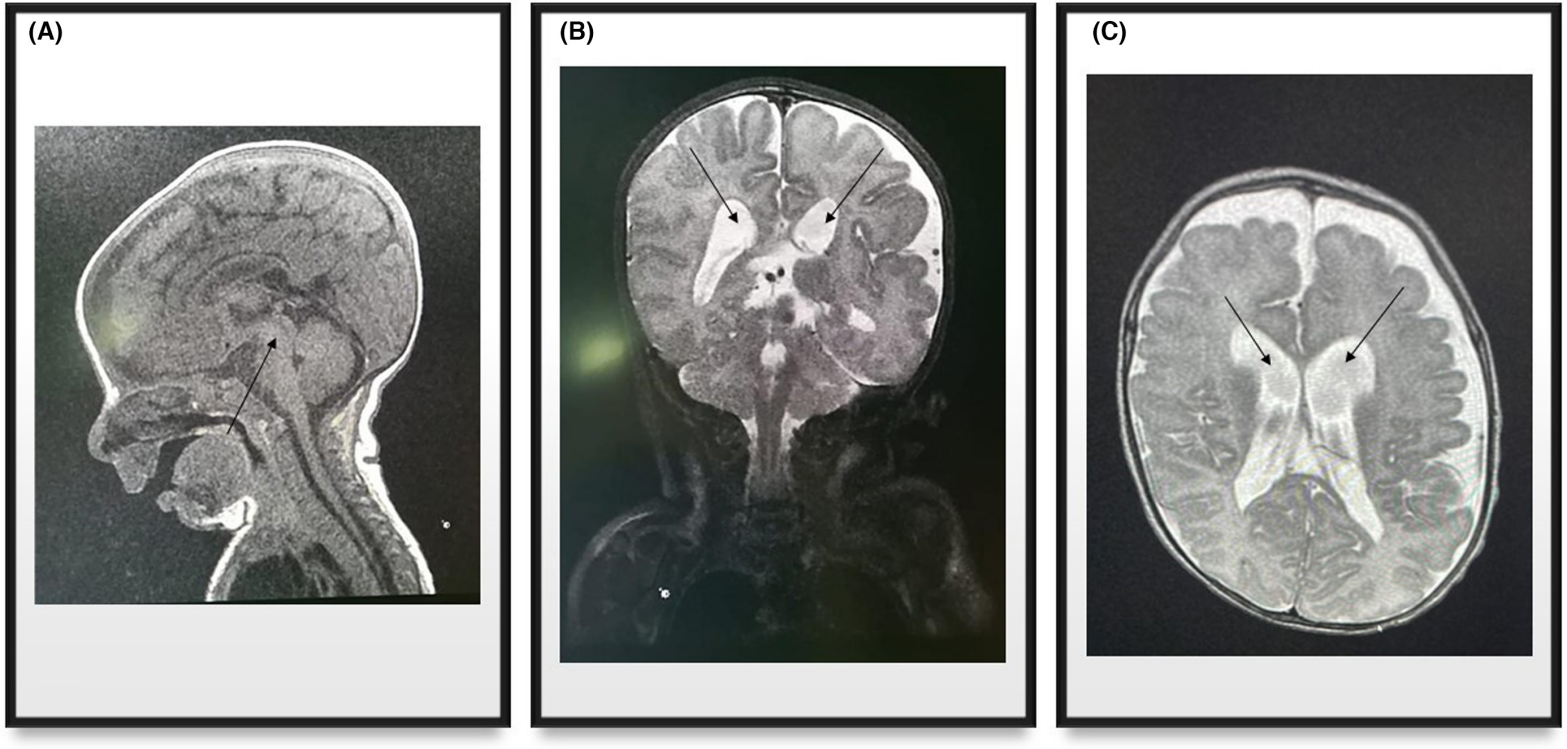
Brain MRI performed at the age of 1 month showing mild dilatation of lateral ventricles
